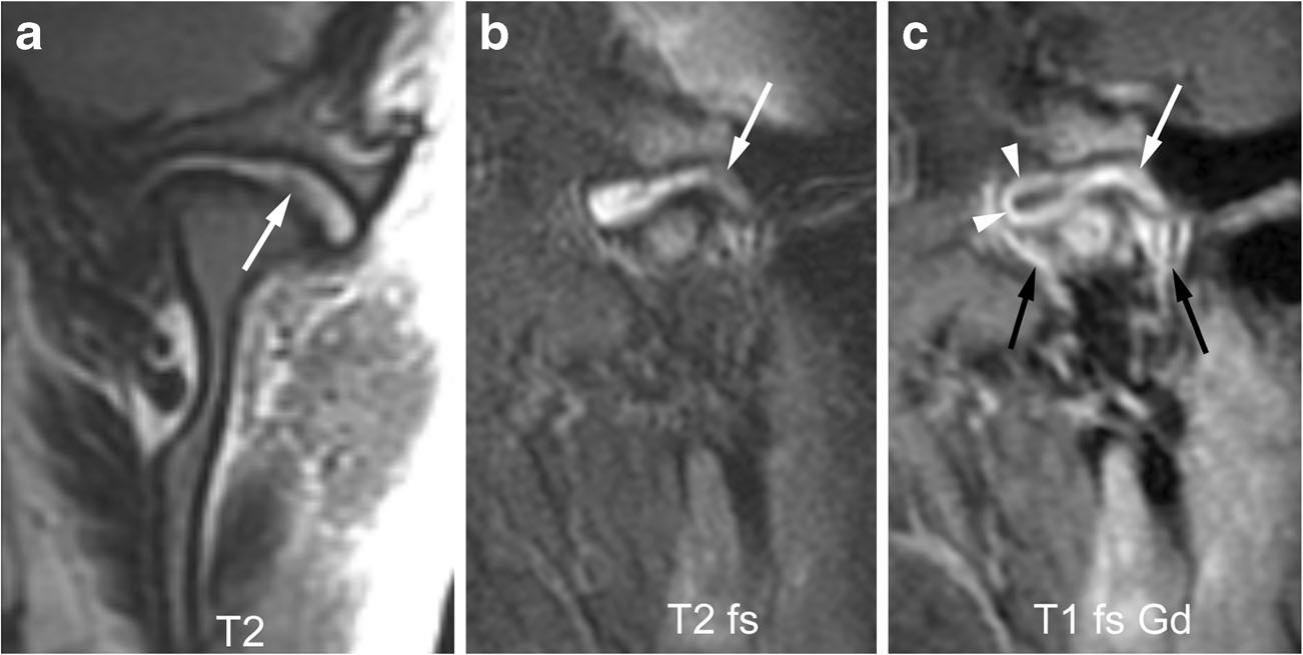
Mild synovial thickening (grade 1) and severe joint enhancement (grade 2). a-b Coronal T2-weighted image (TR/TE 2,600/110 ms, a) and sagittal oblique fat-saturated T2-weighted image (TR/TE 5,400/77 ms, b) show a large effusion with high signal intensity and some nodular areas of synovial thickening with intermediate signal intensity in posterior portion of the upper joint compartment (arrows). c Corresponding early postcontrast fat-saturated T1-weighted (TR/TE 670/10 ms) image demonstrates enhancement of the synovium (white arrow) and peripheral joint fluid (arrowheads) involving the entire upper compartment, with same signal intensity as surrounding veins (black arrows). T1 fs Gd contrast-enhanced fat saturated T1-weighted, T2 T2-weighted, T2 fs fat saturated T2-weighted
